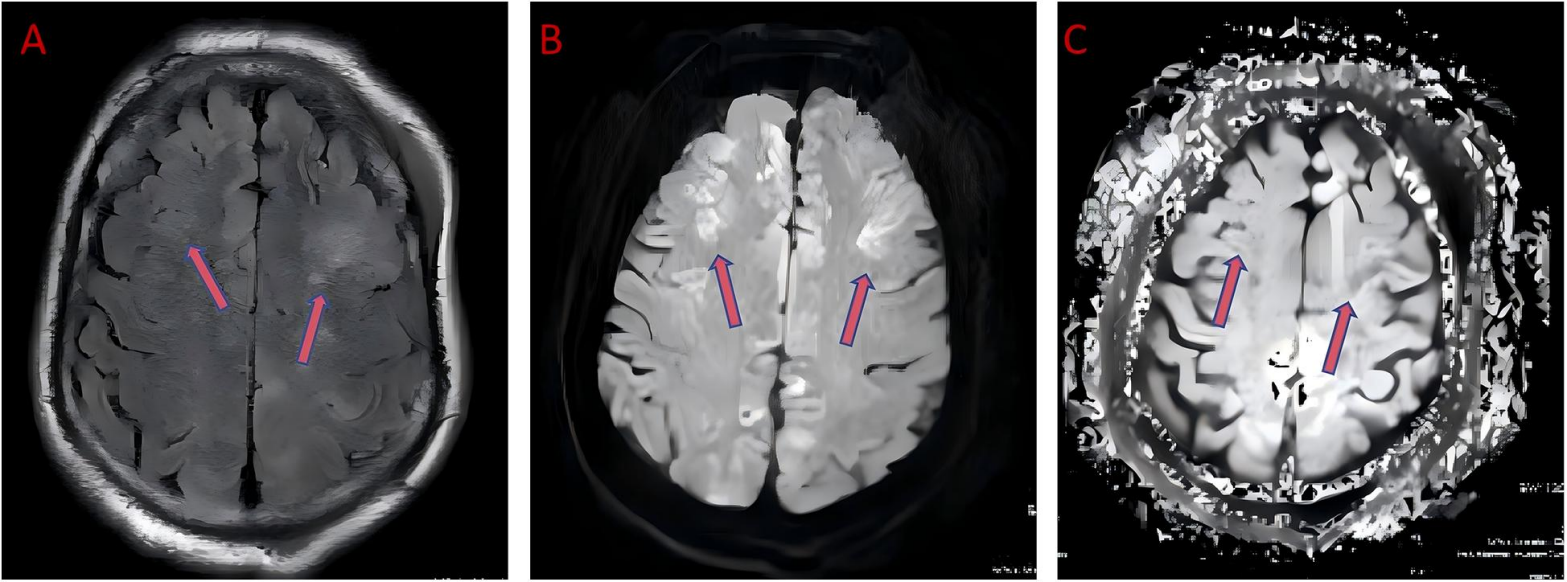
Head MRI shows swelling in the frontal and parietal lobes, indicating ischemic hypoxic encephalopathy. FLAIR imaging shows that brain edema is located in both frontal and parietal lobes, indicating the extent of cerebral ischemia and hypoxia (A),The high signal of DWI sequence indicates ischemic areas in brain tissue (B),The dark signal of the ADC sequence confirms the location of the ischemic and hypoxic regions in the brain (C).
